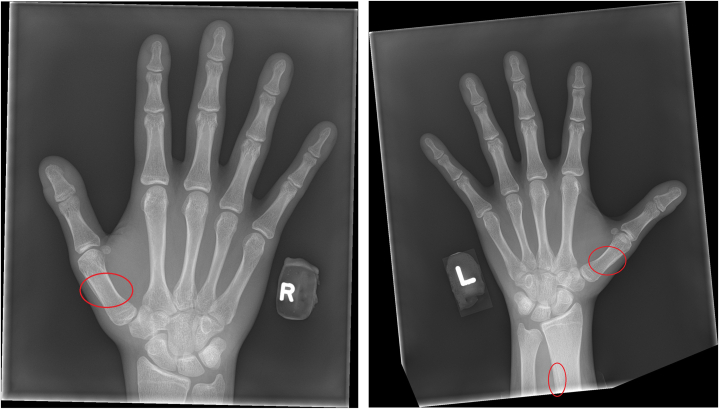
Xrays of the hands of Patient 1. Mild periosteal reactions along the shafts of the bilateral first metacarpal bones and the left distal radius, characteristic of PDP, are *circled*. *PDP*, Pachydermoperiostosis.
